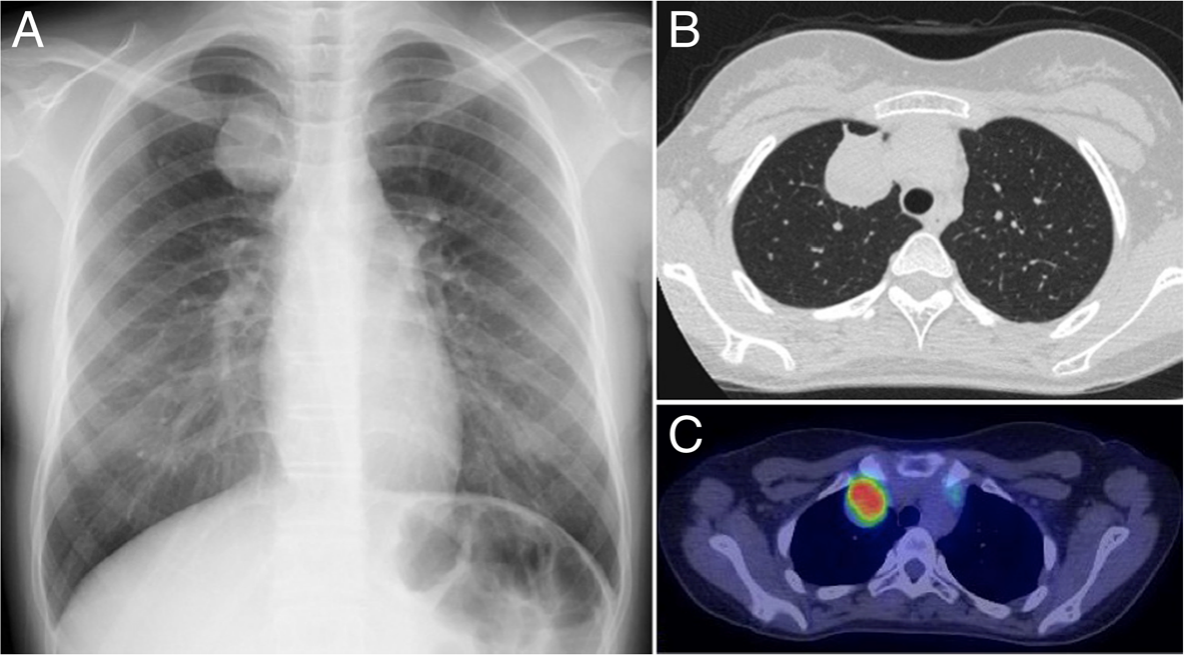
Radiological findings of pulmonary metastasis of osteosarcoma. (A) Chest X-ray shows an abnormal shadow in the right upper lung field. (B) Chest CT reveals a 35-mm tumor with a relatively smooth surface in right S1. (C) PET shows FDG uptake by the tumor.
